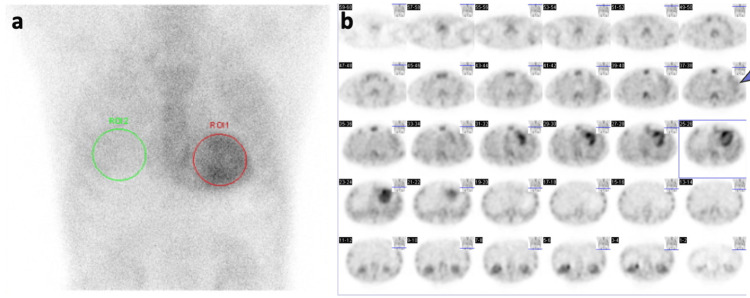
99mTechnetium pyrophosphate scintigraphy of the patient. (a) Anteroposterior scintigraphy view displaying cardiac uptake. The red circle delineates the myocardial region, and the green circle demarcates the contralateral reference area. (b) Sequential axial slices of ^99m^Technetium pyrophosphate scintigraphy performed one hour post-injection. An arrowhead highlights the notable radiotracer uptake in the patient's left upper pulmonary lobe.
